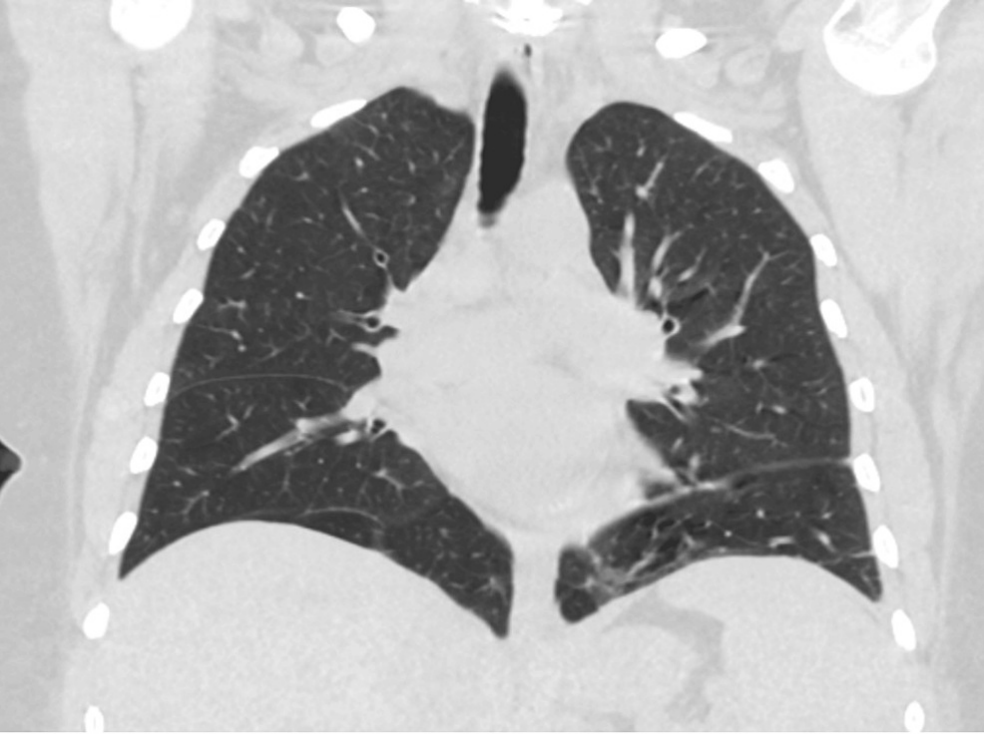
CT of the chest without any pathology CT: computed tomography
